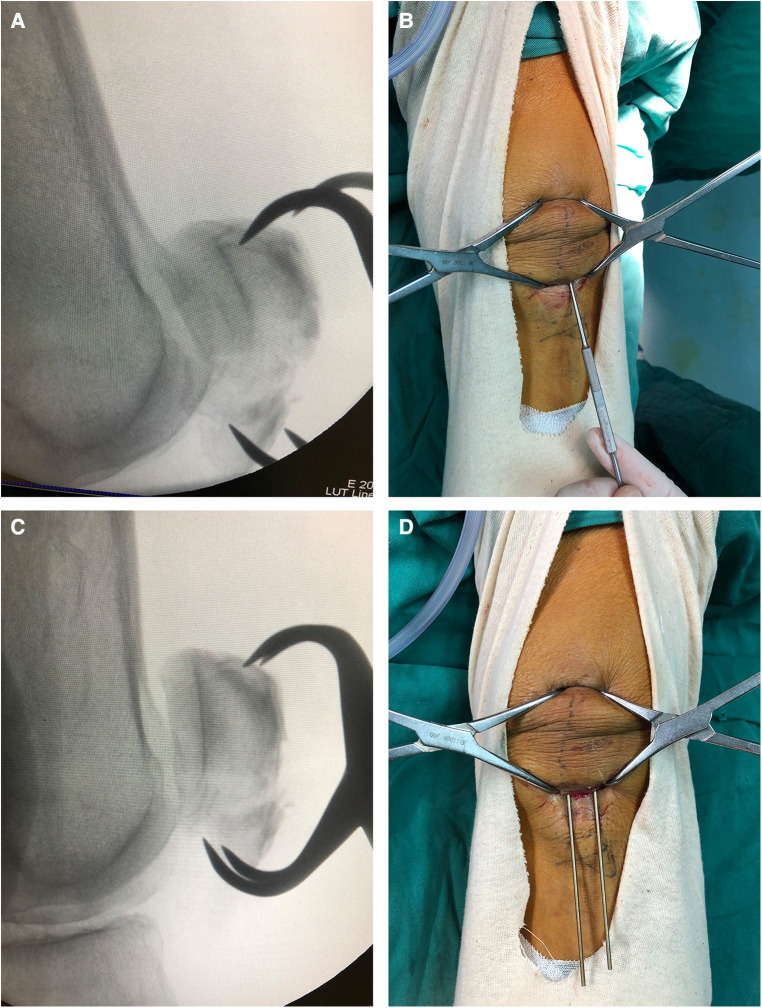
Joint application of periosteal elevator and pointed bone forceps helps achieve bone fracture reduction. (A) Radiograph taken after the initial reduction procedure reveals that fracture reduction was not achieved as the articular step-off still presented. (B) Photograph shows that a small periosteal elevator is passed through the patellar tendon to reach the articular surface of the distal bone fragments and elevate it, and then, two-pointed bone forceps were utilized to close the fracture gap. (C) Radiograph taken after the reduction procedure confirmed that the fracture was reduced. (D) Photograph shows two k-wires passing through the fracture to maintain the fracture reduction.
